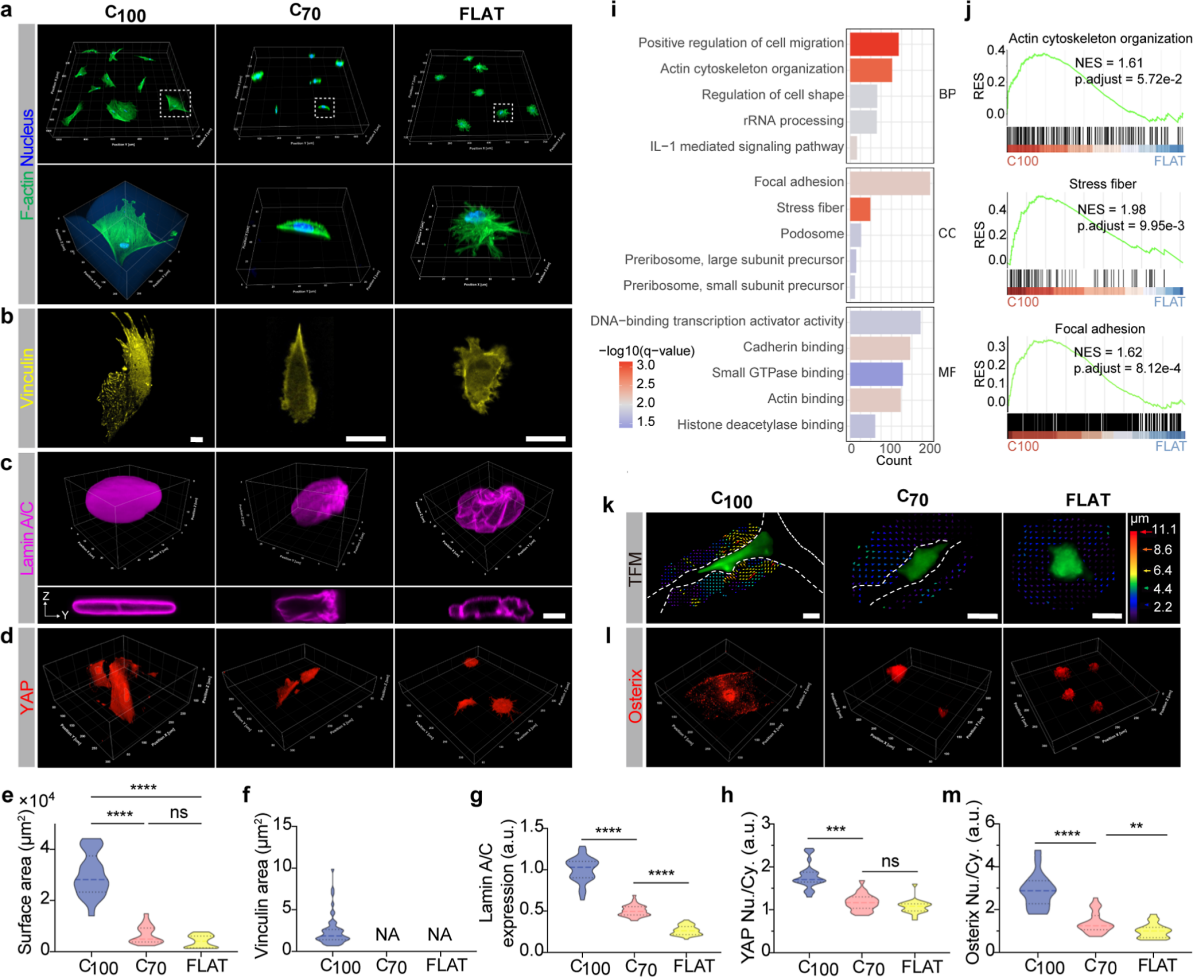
Multiaxial curvature on ultrasoft substrate
